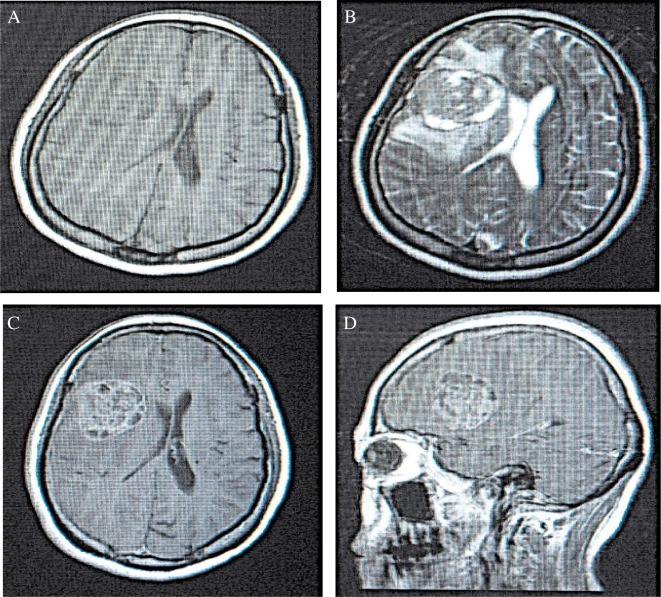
The MRI of a high grade glioma. A: A heterogeneous and slightly long T1 signal with unclear border was detected in the T1WI sequence at the transverse plane in the left frontal lobe. B: A heterogeneous and slightly long T2 signal with unclear border was observed in the T2WI sequence at the transverse plane in the left frontal lobe. An edema band existed around the tumor. C: A T1WI enhancement scan at the transverse plane showed a wreath-like and heterogeneous enhancement. D: A T1WI enhancement scanning at the sagittal plane showed a wreath-like and heterogeneous enhancement.
